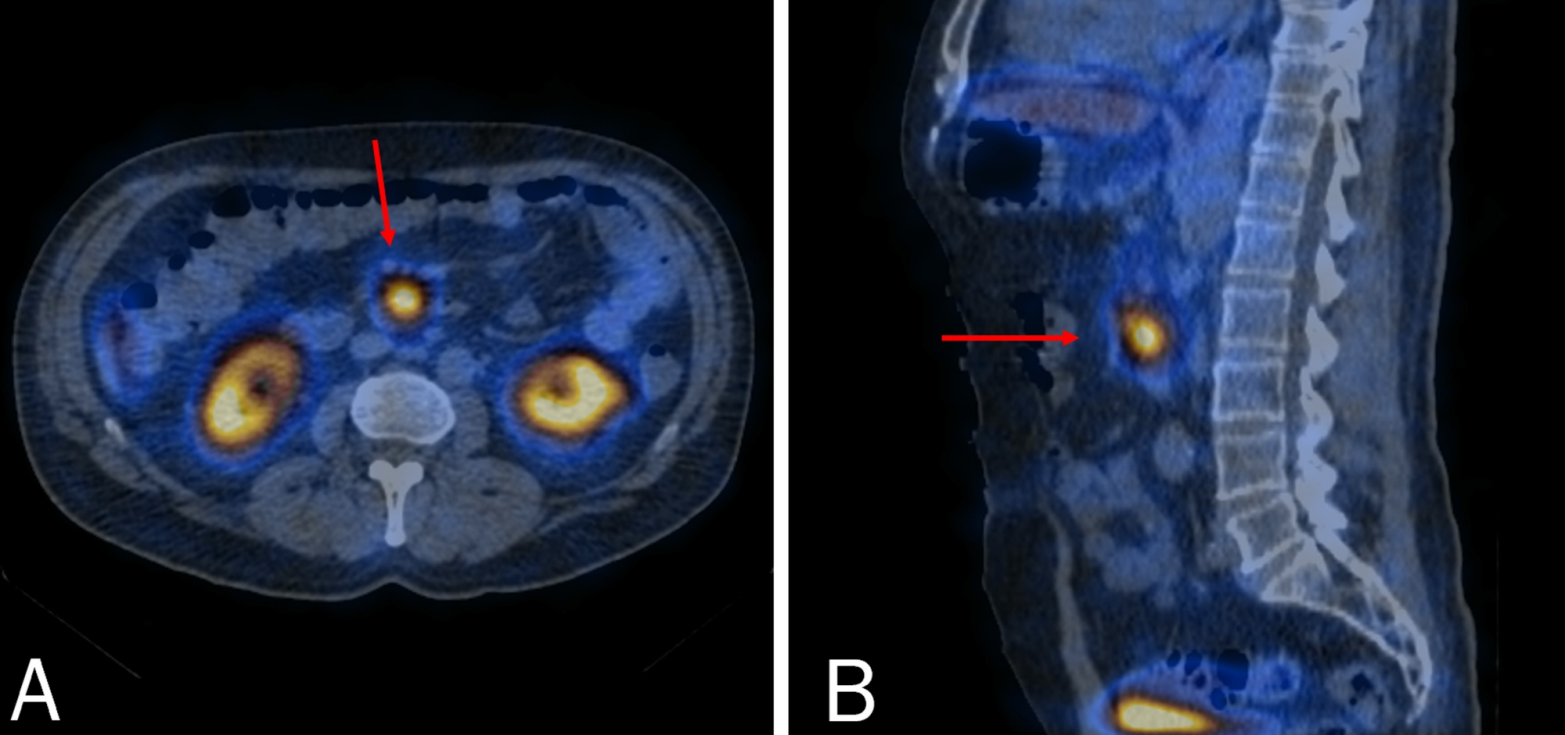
SPECT: transverse (A) and sagittal (B) views demonstrating focal uptake of contrast (arrows) behind the head of the pancreas, adjacent to the third portion of the duodenum. SPECT: single-photon emission computerized tomography.
